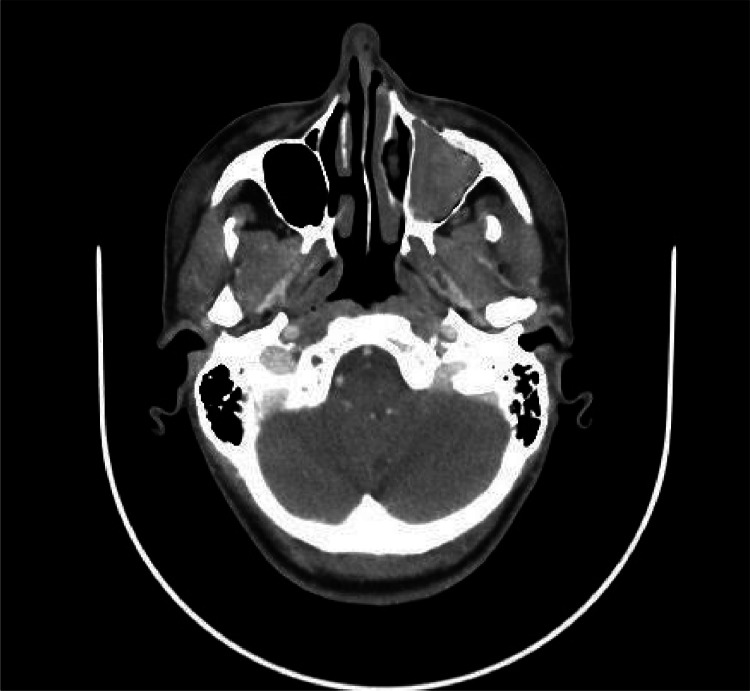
An axial CT image of the skull showed the left maxillary sinus obliterated.
